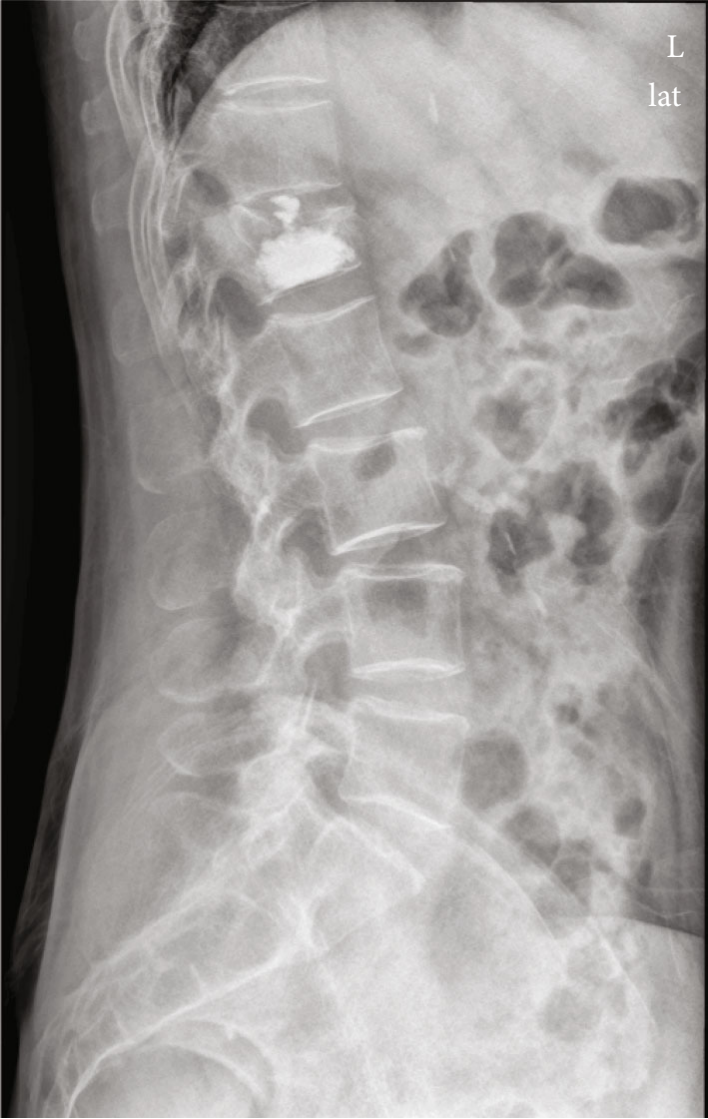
Cement leakage into the intervertebral disc on direct postoperative radiograph.
